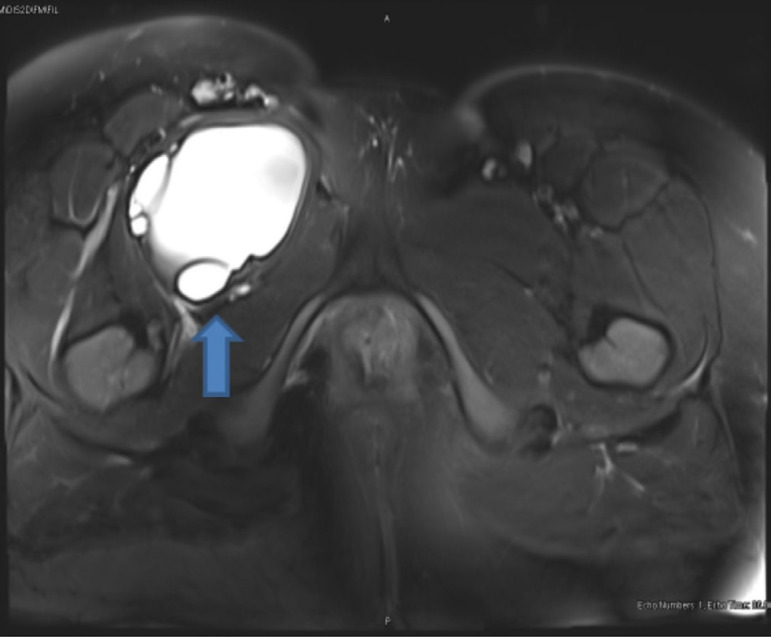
MRI image of pelvic iliofemoral hydatid cyst.
